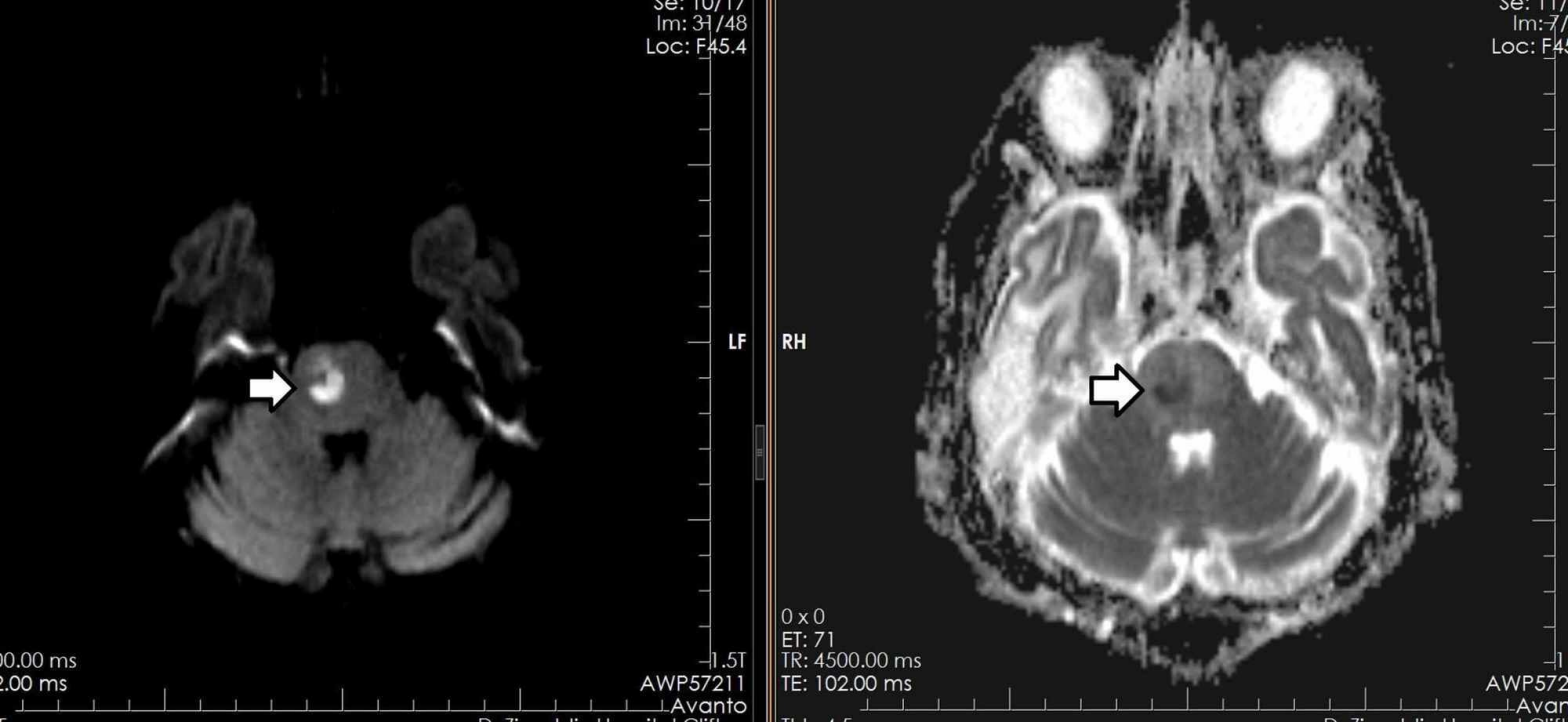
Similar patient with a right hemipons abscess showing diffusion restriction on DWI and ADC sequences DWI: diffusion-weighted magnetic resonance imaging; ADC: apparent diffusion coefficient
